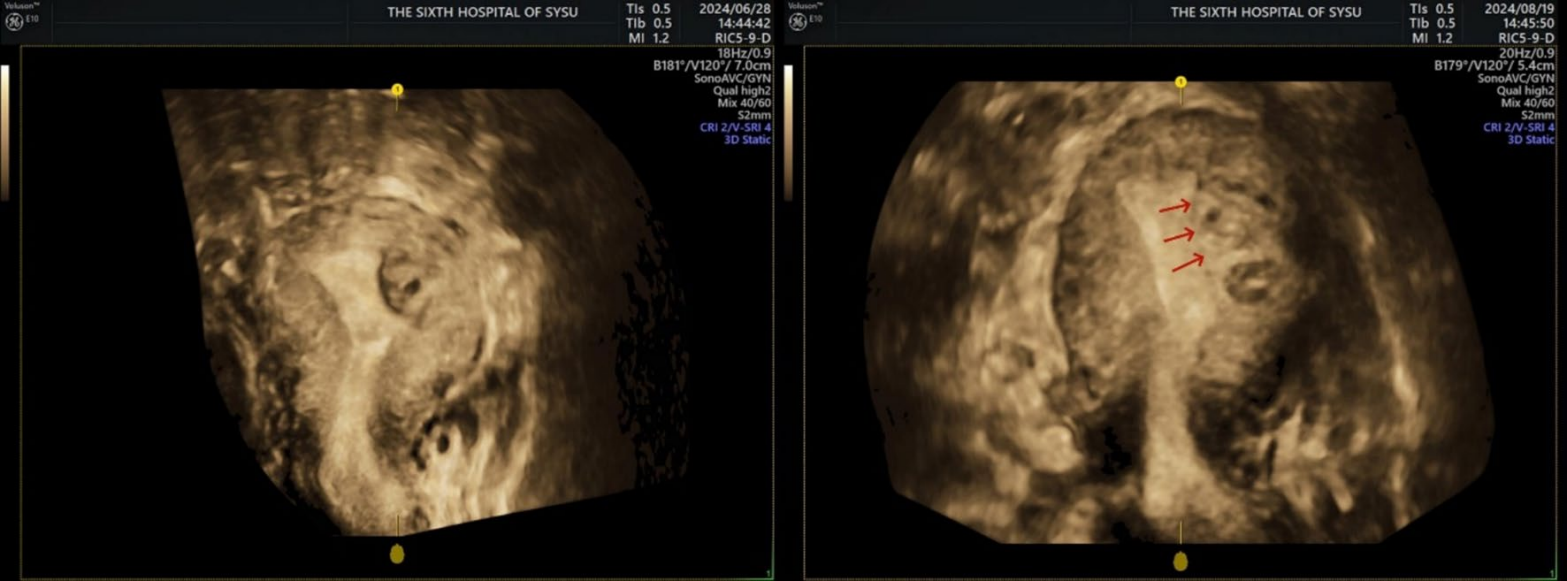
Three-dimensional ultrasound images of before and after electrotomy for hysteroscopic myomectomy, arrow pointing to residual fibroids
